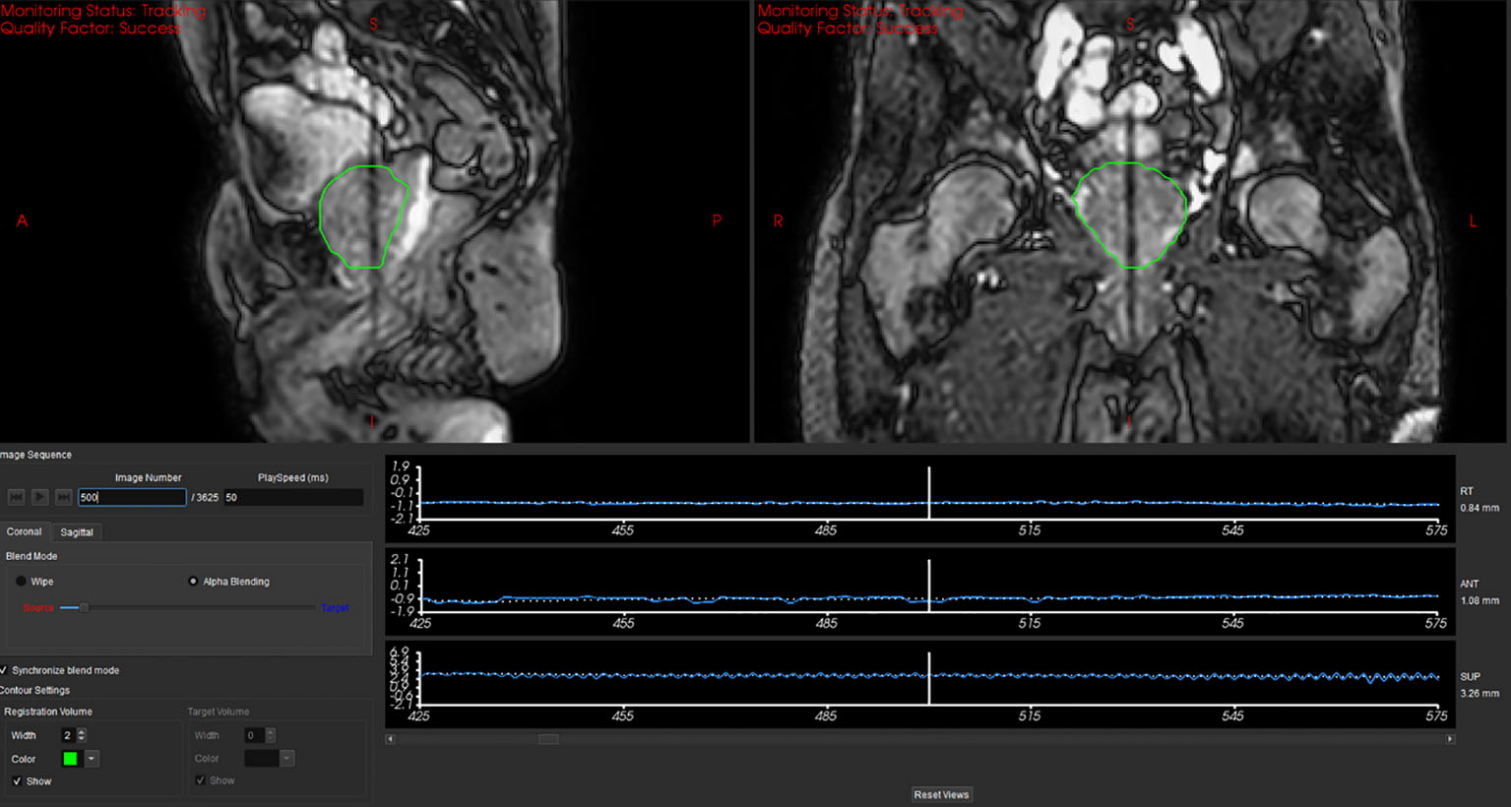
Screen Capture depicting the motion tracking software interface. The green contour represents the position of the prostate as identified by the tracking algorithm in the live cine MRI in a sagittal and coronal plane. The bottom of the image depicts the tracked position of the prostate in the right/left, ant/post, and sup/inf positions on the current frame as well as history of previous tracked positions.
